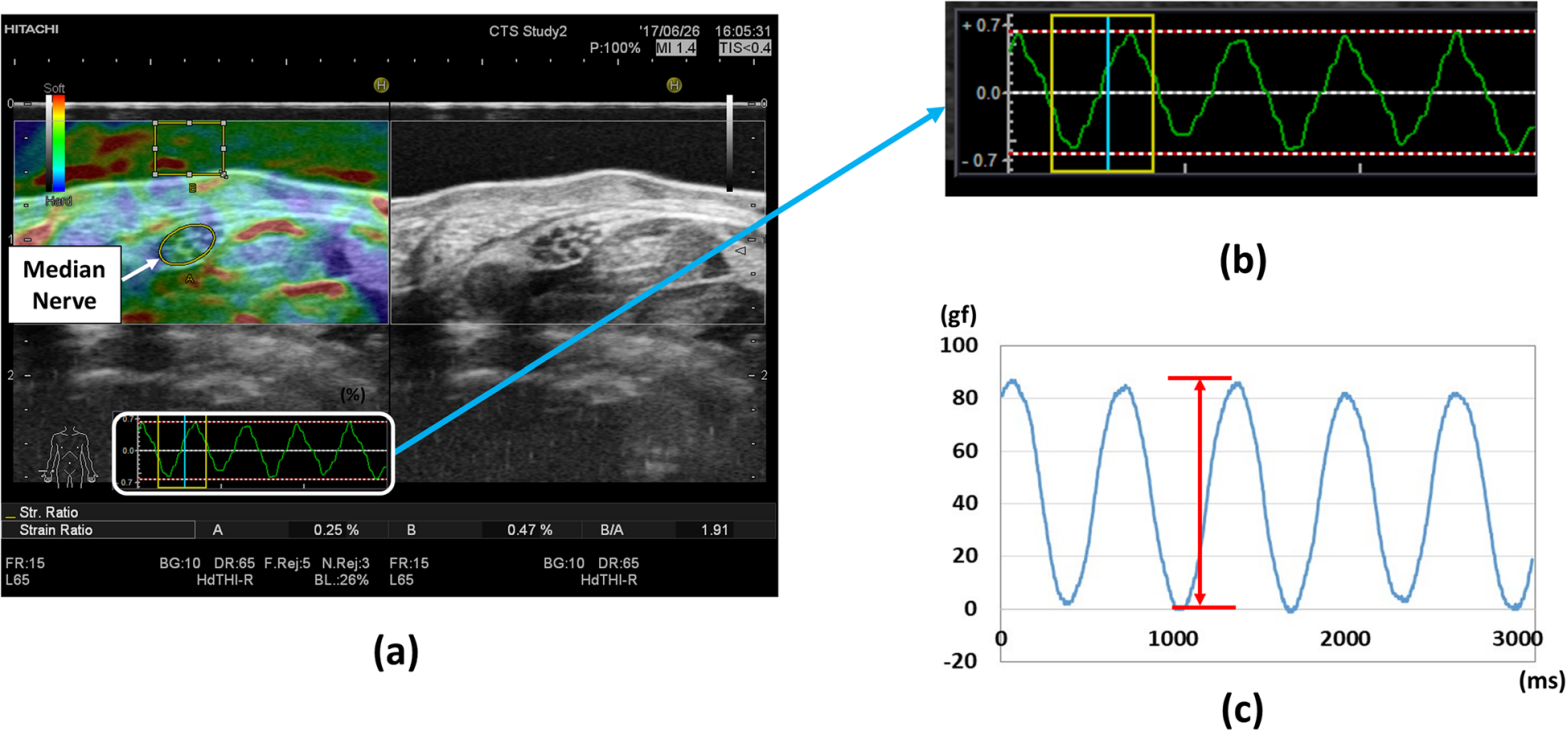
An example image of strain and pressure measurement. a Real-time Tissue Elastography mode of the ultrasound system. b An example of a strain waveform. c An example of a pressure waveform
